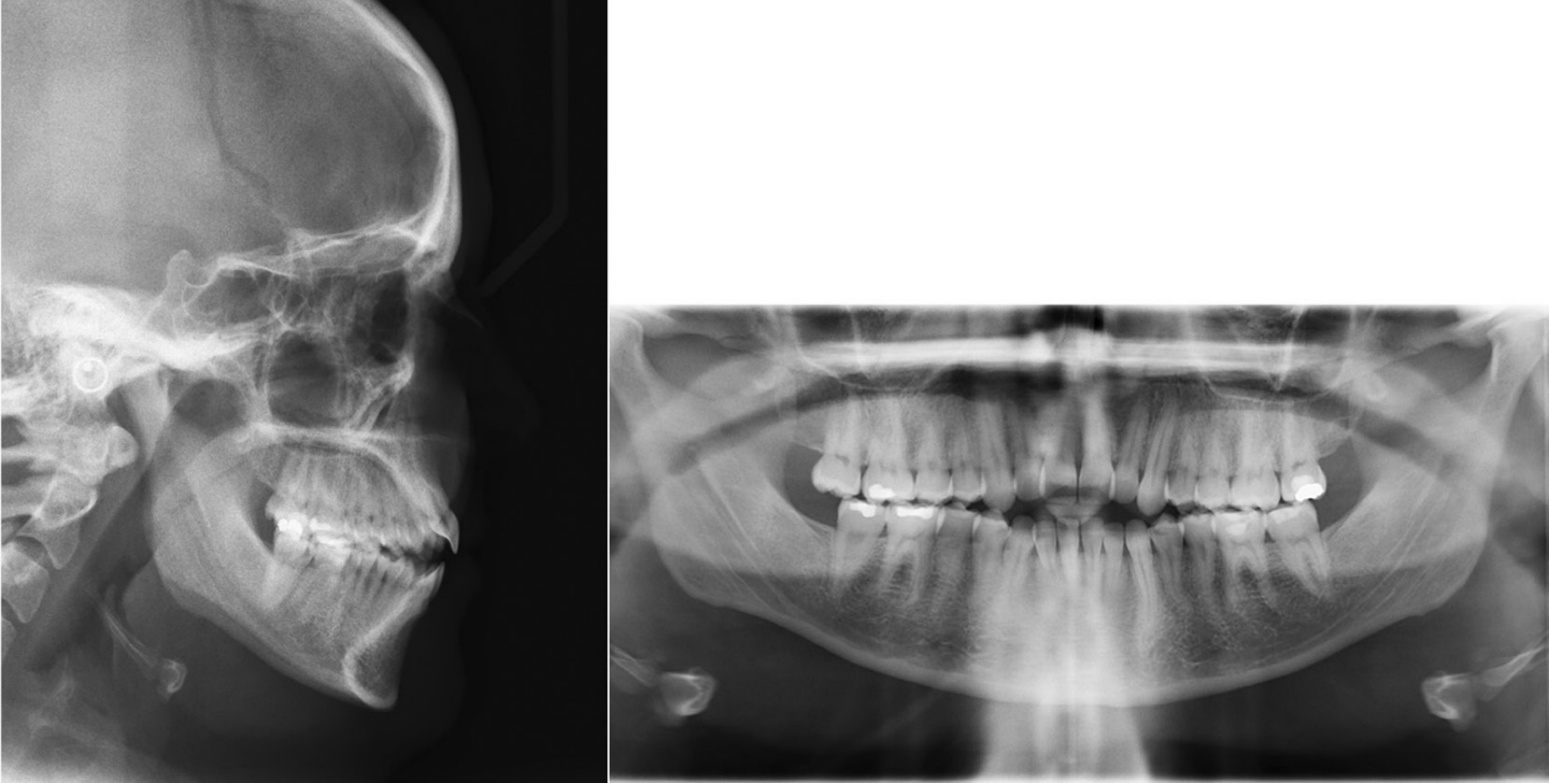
Pre‐treatment radiographs
